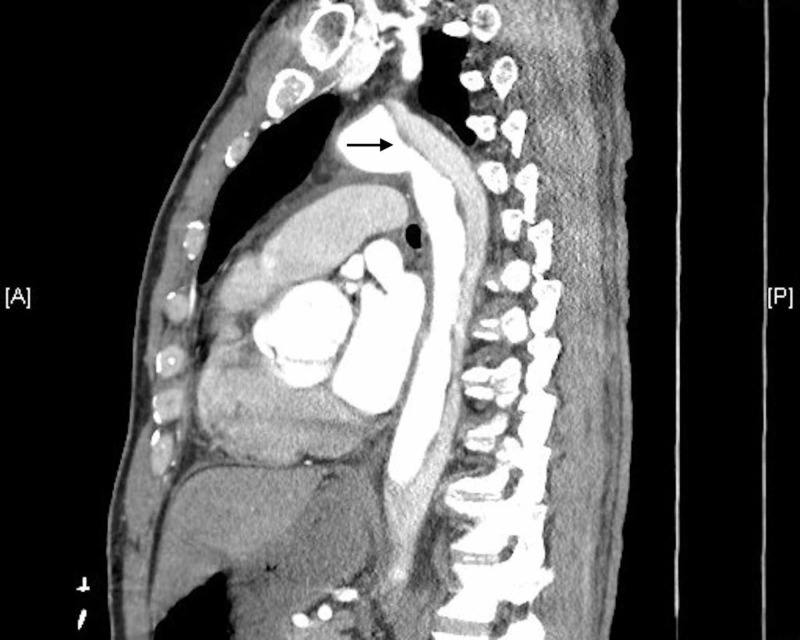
Sagittal CT view of aortic dissection CT, computed tomography
